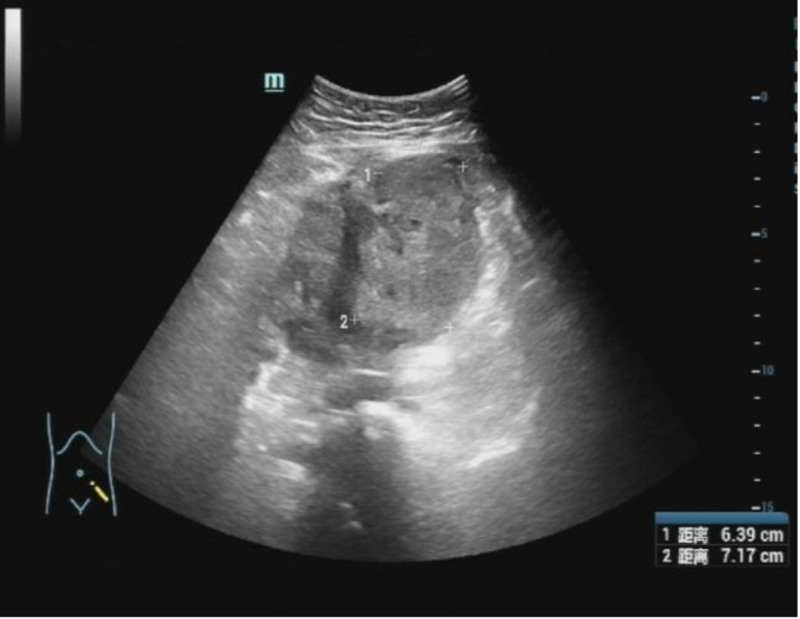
Huge hematoma was observed in the ultrasonography.
